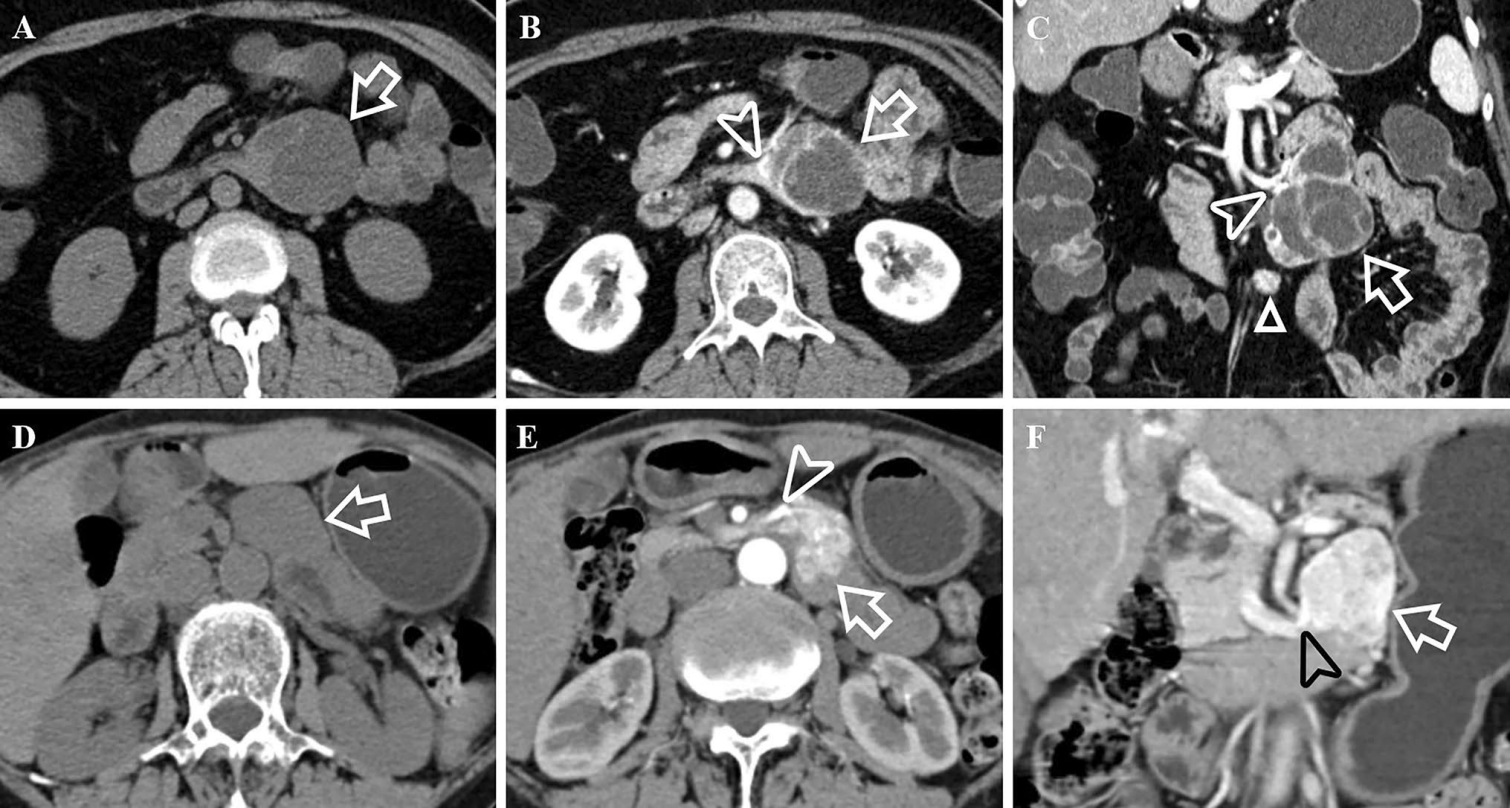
62-year-old female with a G1 dNEN. Axial image in the unenhanced phase (A), and axial (B), coronal (C) images in the portal venous phase show a well-defined, irregular lobulated, complex cystic lesion (white arrows) with extraluminal growth pattern in the ascending part of the duodenum. White arrowheads show that it drains to superior mesenteric vein. The feeding arteries are very slender and are therefore not shown. CT attenuation value of tumor’s solid component in the unenhanced phase is 41.85 HU. Besides, there is also a hypervascular nodule in the mesoduodenum below it (white triangle). 76-year-old female with a dGIST. Unenhanced image (D) and enhanced images in the arterial phase (E) and portal venous phase (F) show a well-defined, irregular, solid lesion (white arrows) with mixed growth pattern in the ascending part of the duodenum. There were abundant intratumoral vessels in the lesion. It is fed by superior mesenteric artery (white arrowhead) and drains to superior mesenteric vein (black arrowhead). CT attenuation value of the tumor in the unenhanced phase is 38.18 HU
